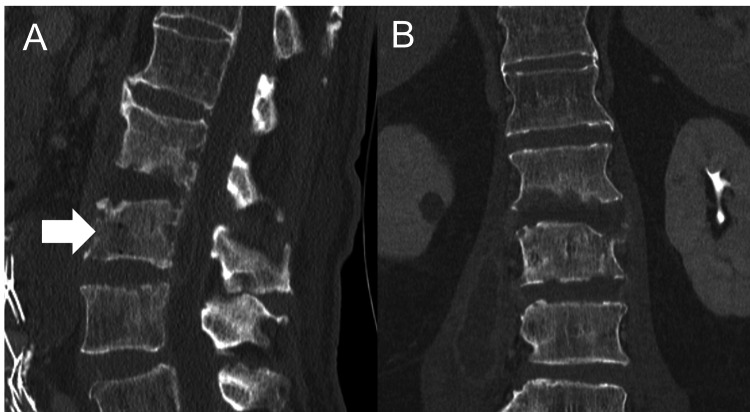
Preoperative computed tomography scan of the lumbar spine (A) sagittal image, (B) coronal image Computed tomography showing bone destruction at the L2 vertebral body and L3 vertebral body showing a gas-forming lesion (arrow).
